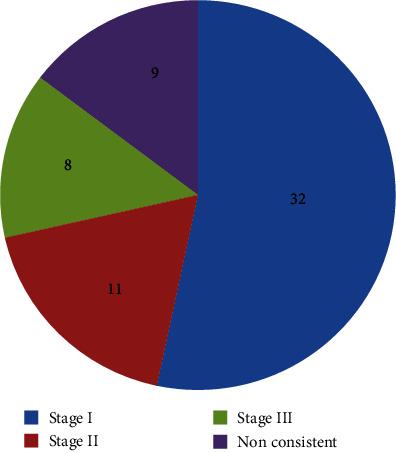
Consistency analysis of high KV DR and CT detection.
